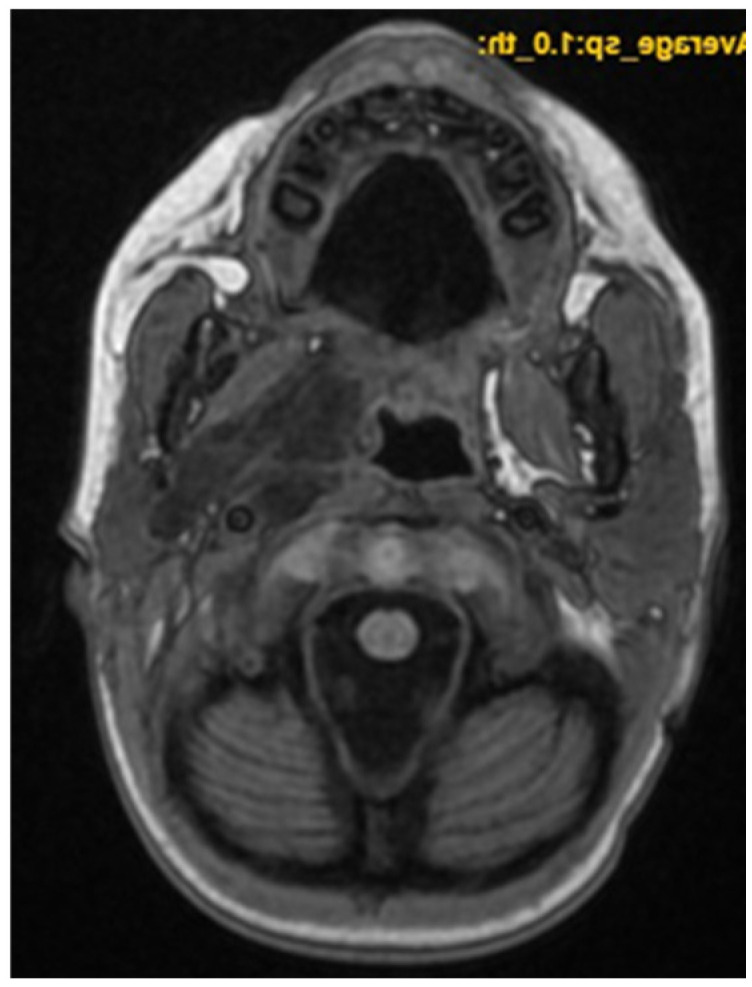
MRI on the 4th month from the start of chemotherapy.
